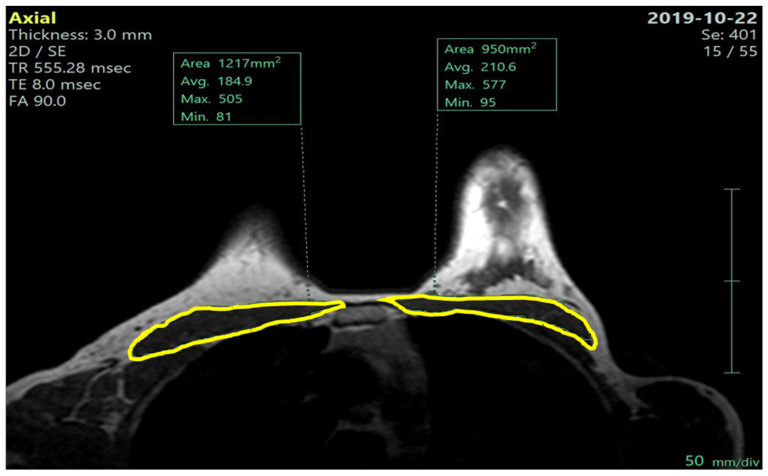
Pre-NACT left and right pectoralis muscle areas (MRI—axial T1 non-fat sat image).
